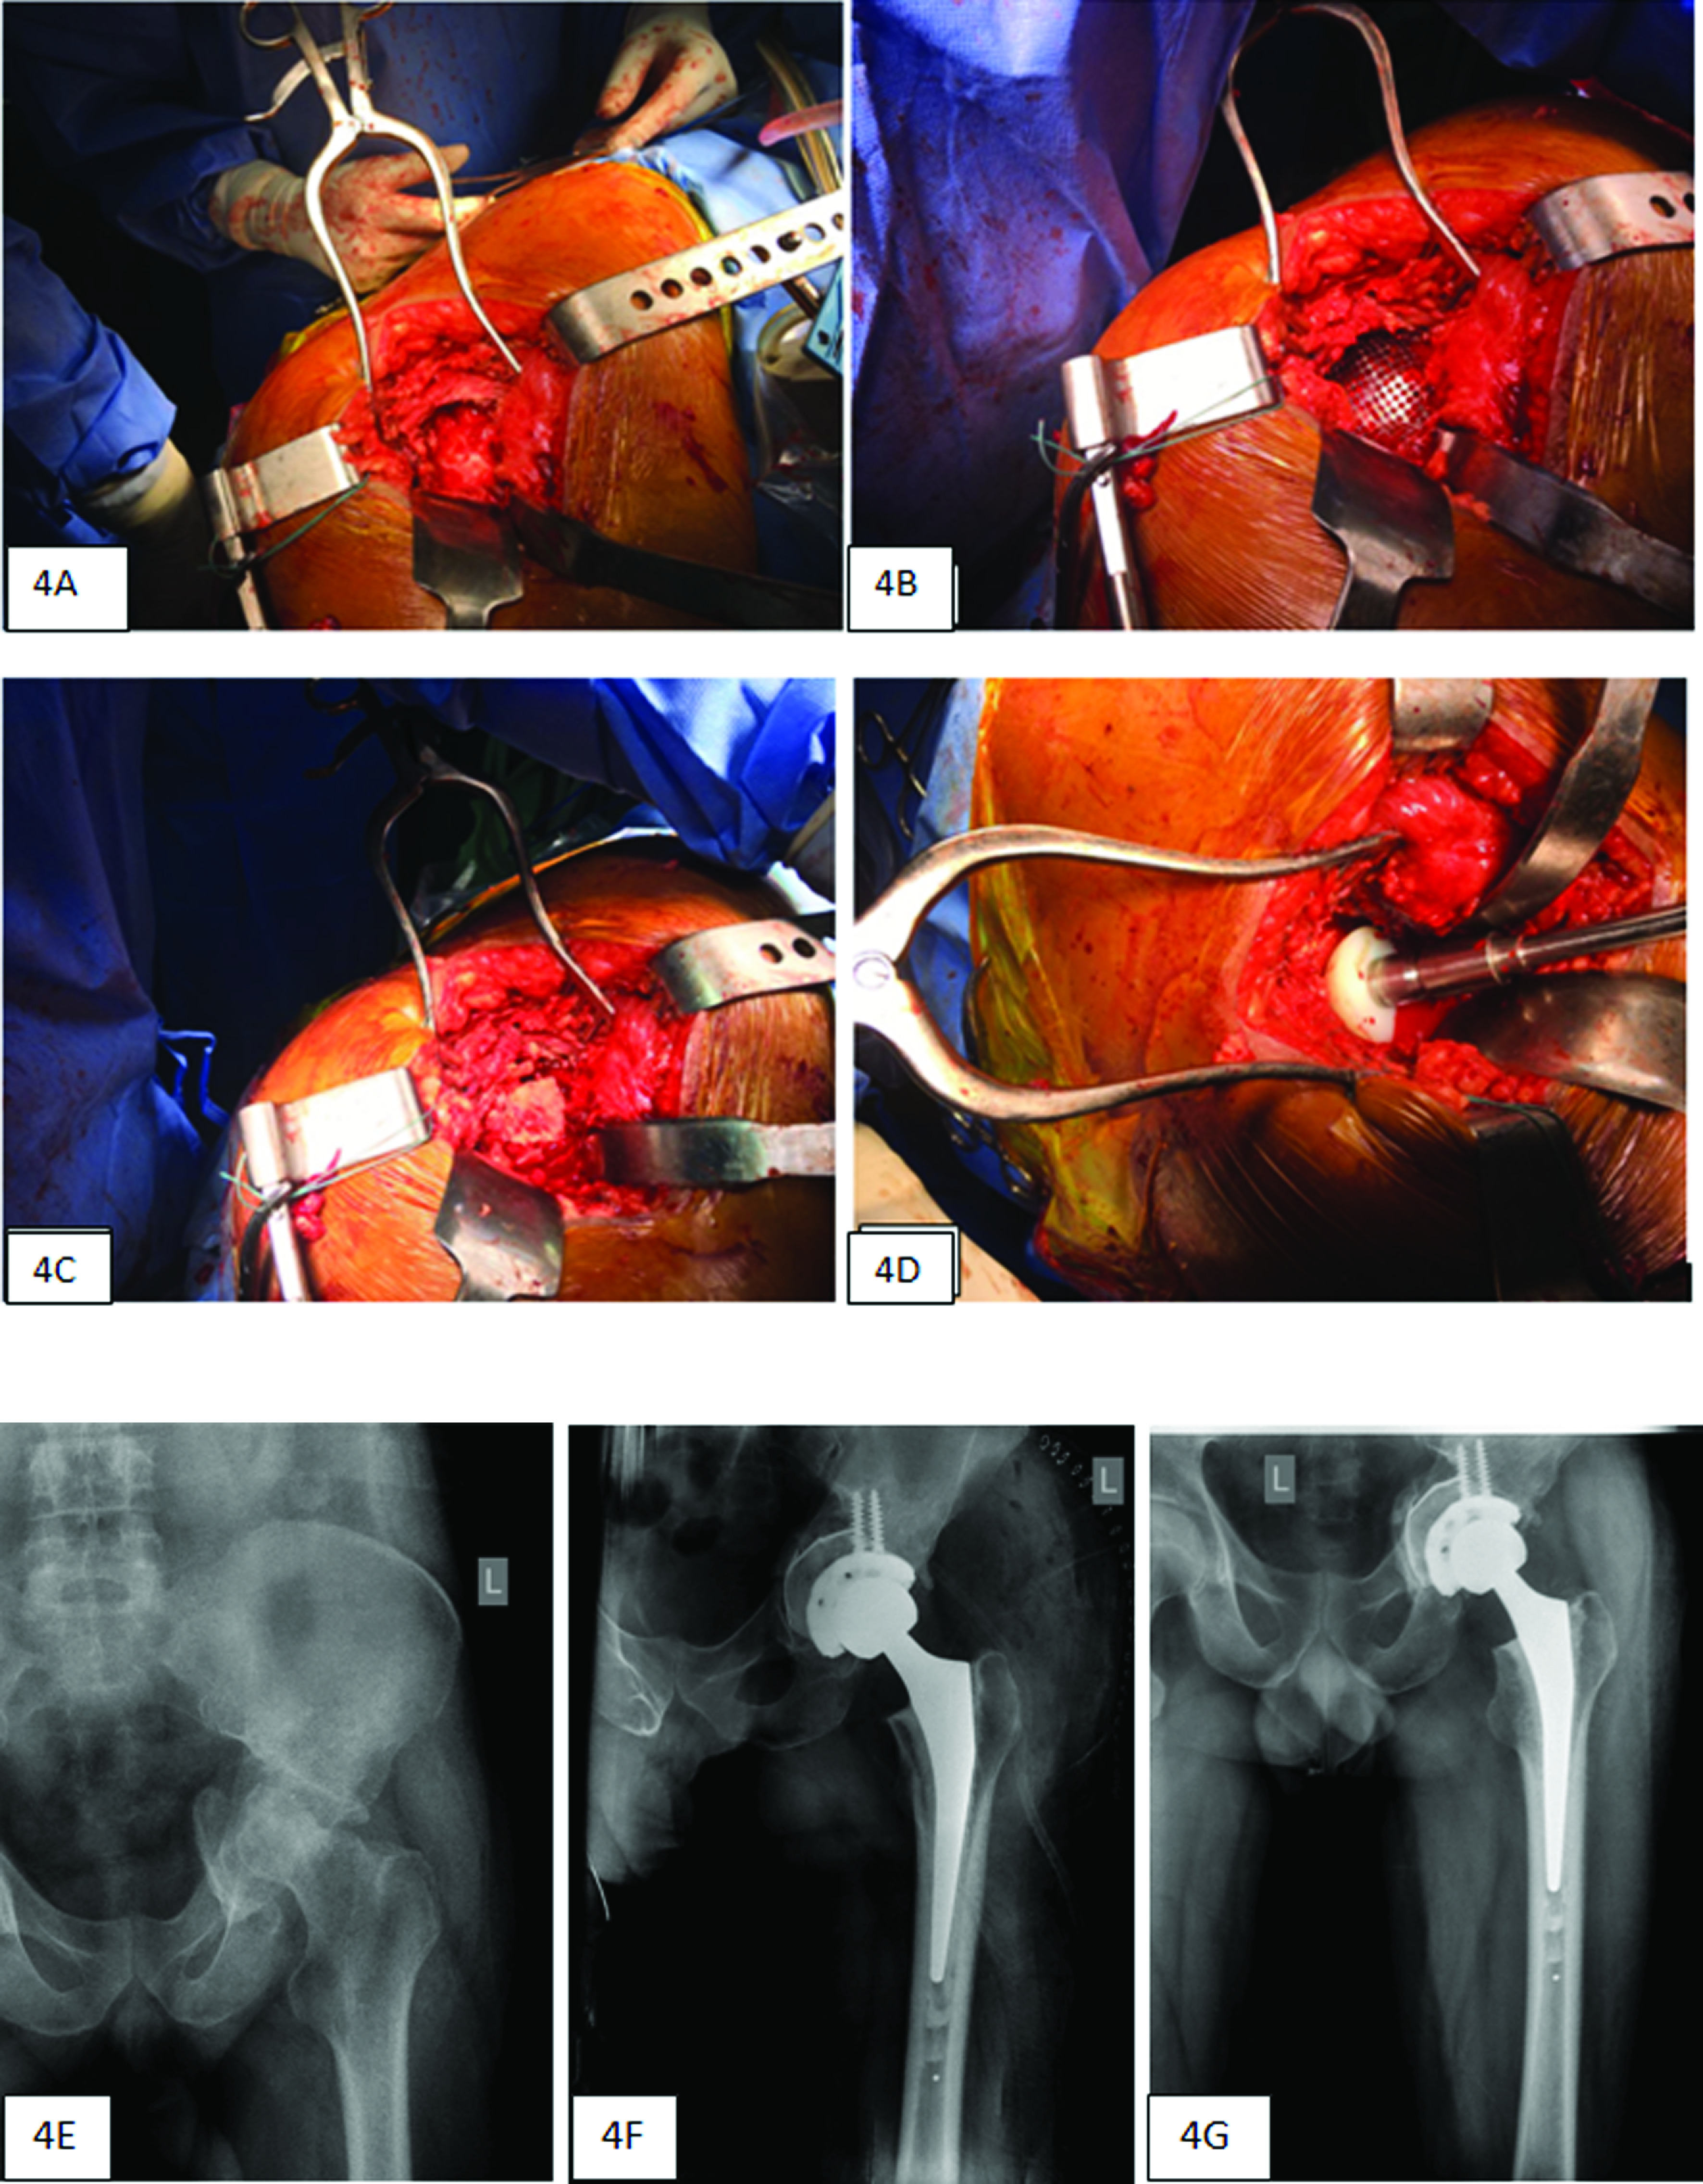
(A) Central defect with no intact medial wall. (B) Reconstruction of the defect with mesh and bone graft. (C) Bone graft over the mesh. (D) Impaction. (E) Radiograph showing a medial wall defect. (F) Radiograph showing medial wall reconstruction with mesh and bone graft. (G) Well-consolidated bone graft at 5-years follow-up.
